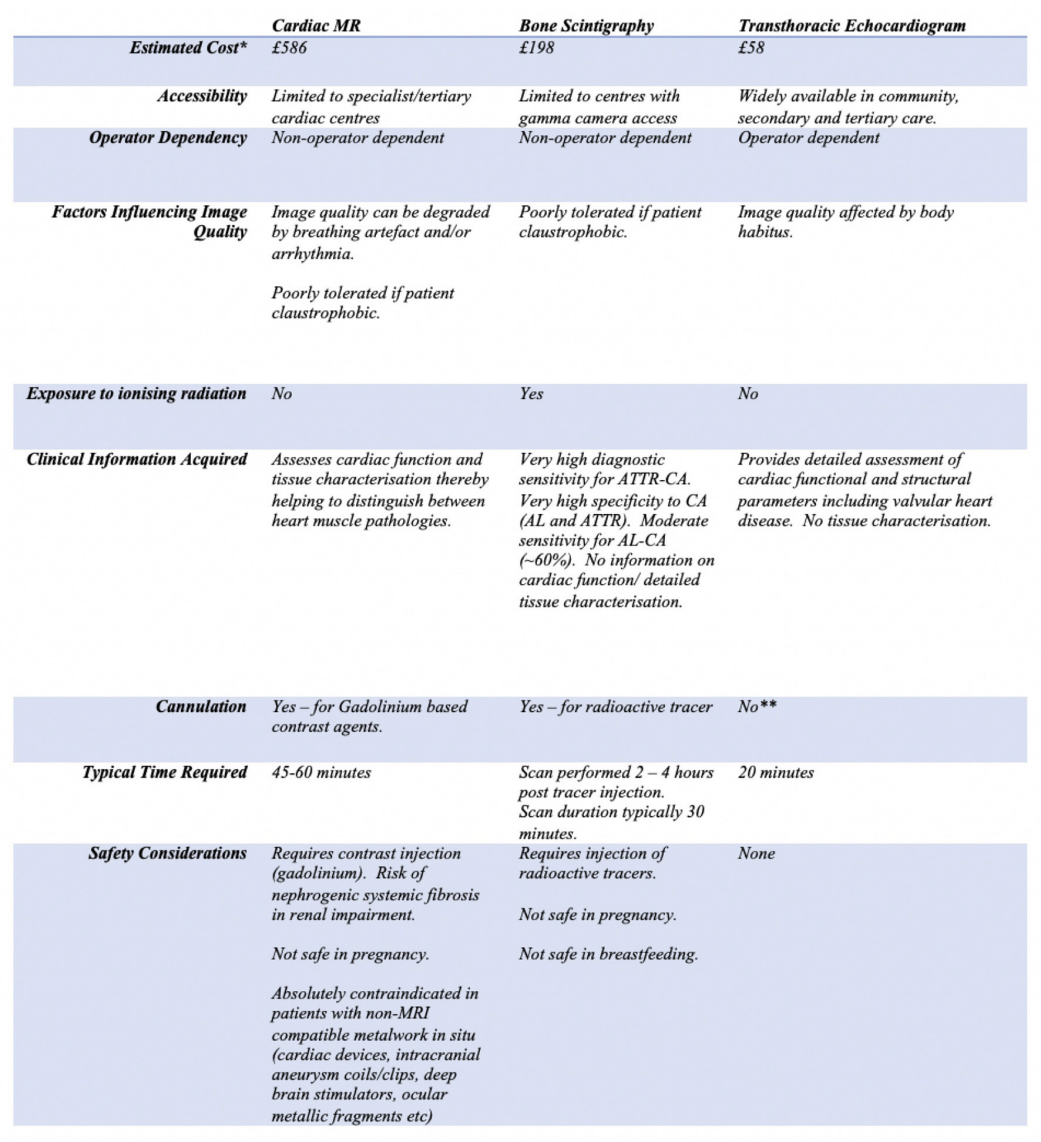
Table highlighting the individual limitations and strengths of cardiac magnetic resonance, bone scintigraphy and echocardiography in the context of assessing patients with cardiac amyloidosis (*costing estimates based on UK National Health Service Tariffs 2020/2021, estimate includes cost of reporting scans, whilst cannulation is required for contrast echocardiography, this is not routinely indicated when assessing for CA).
